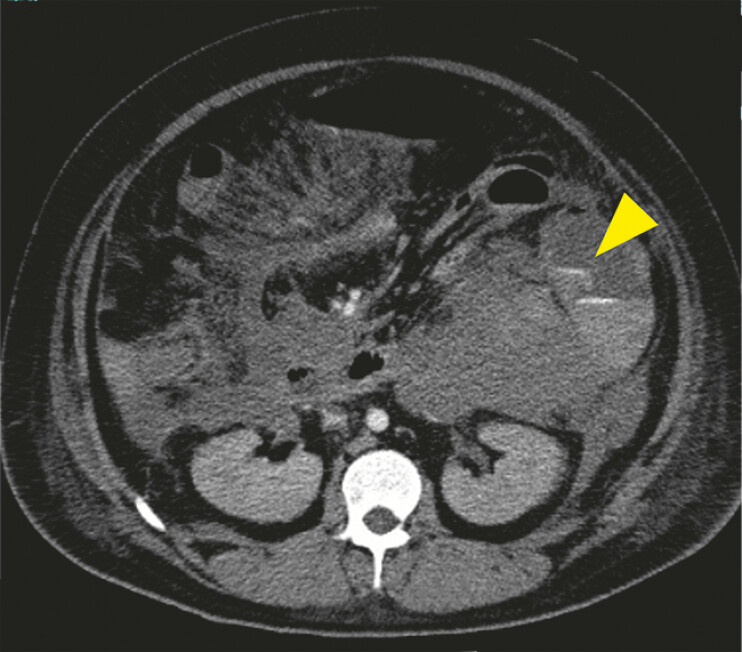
Contrast-enhanced computed tomography demonstrated a ruptured left colic artery pseudoaneurysm. Active extravasation (arrowhead) from the aneurysm is visible, with retroperitoneal hematoma.
